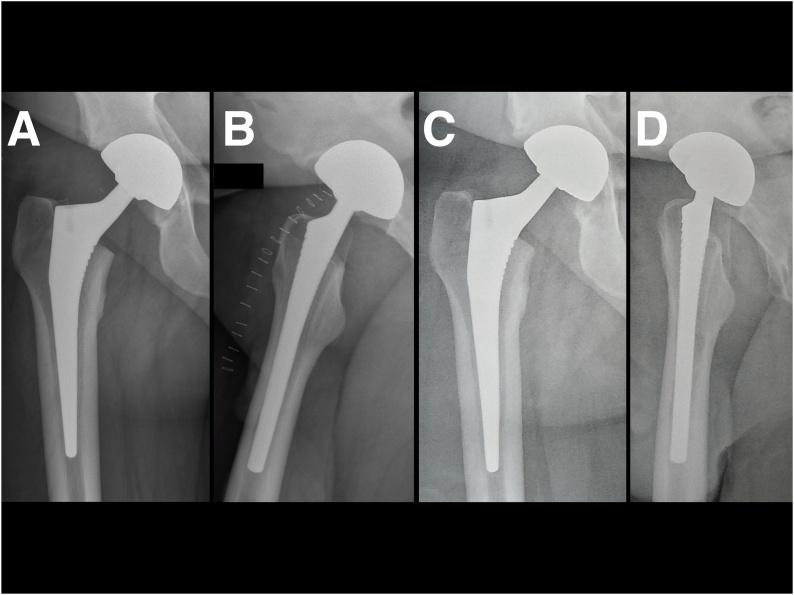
Immediate postoperative X-rays of the right hip after total hip arthroplasty (2A: AP view; 2B: profile view), and X-rays taken at the 12-month follow-up showing osseointegration without loosening (C: AP view; D: profile view).
